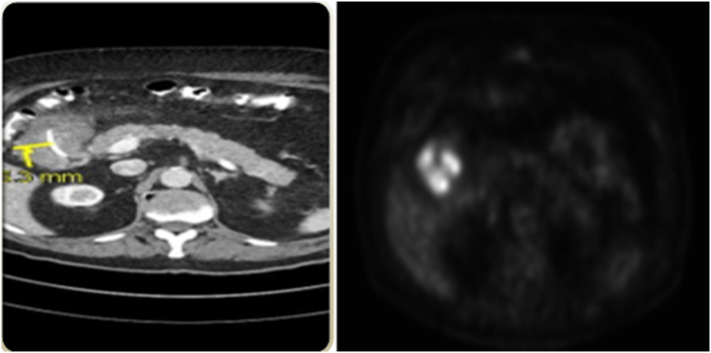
Radiologic view of the tumor a) Enhanced abdominal CT-scan: suspicious abdominal mass in the pyloric region b) Positron Emission Tomography – Computed Tomography: Hyper metabolic abdominal mass in the pyloric region, highly suspicious of neoplasia.
